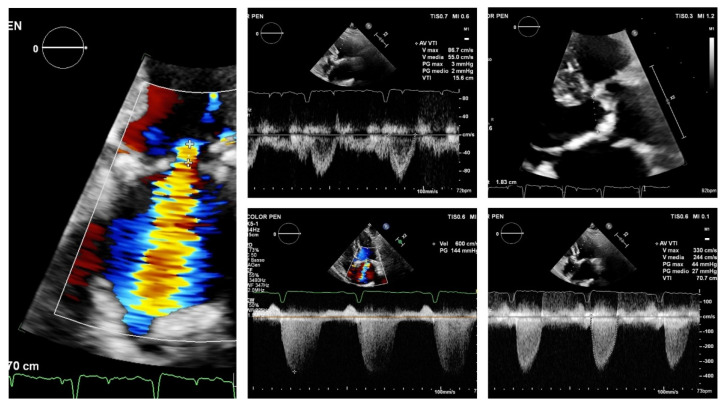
An elderly woman with low-gradient severe AS associated with MR and preserved ejection fraction. The MPG is 27 mm Hg and the Vmax is 330 cm/s. The left ventricular outflow tract’s pulsed wave Doppler velocity-time integral and diameter are 15.6 cm and 1.8 cm, respectively. The calculated SVI is 40 mL (24 mL/m^2^) and AVA is 0.58 cm^2^. The EROA by the 2D-PISA method is 0.20 cm^2^, Rvol = 37 mL. Of note, the MR jet velocity is 6 m/s because jet momentum flux (which is flow • v or EROA • v2) determines the color Doppler jet area. A 6 m/s jet shows 44% larger than a 5 m/s jet through the same EROA. AS: aortic stenosis; MR: mitral regurgitation; MPG: mean transaortic pressure gradient; Vmax: maximal transaortic velocity; SVI: stroke volume index; AVA: functional aortic valve area; v: velocity. 2D: bi-dimensional echocardiography; PISA: proximal isovelocity surface area; RVol: regurgitant volume; EROA: effective regurgitant orifice area.
